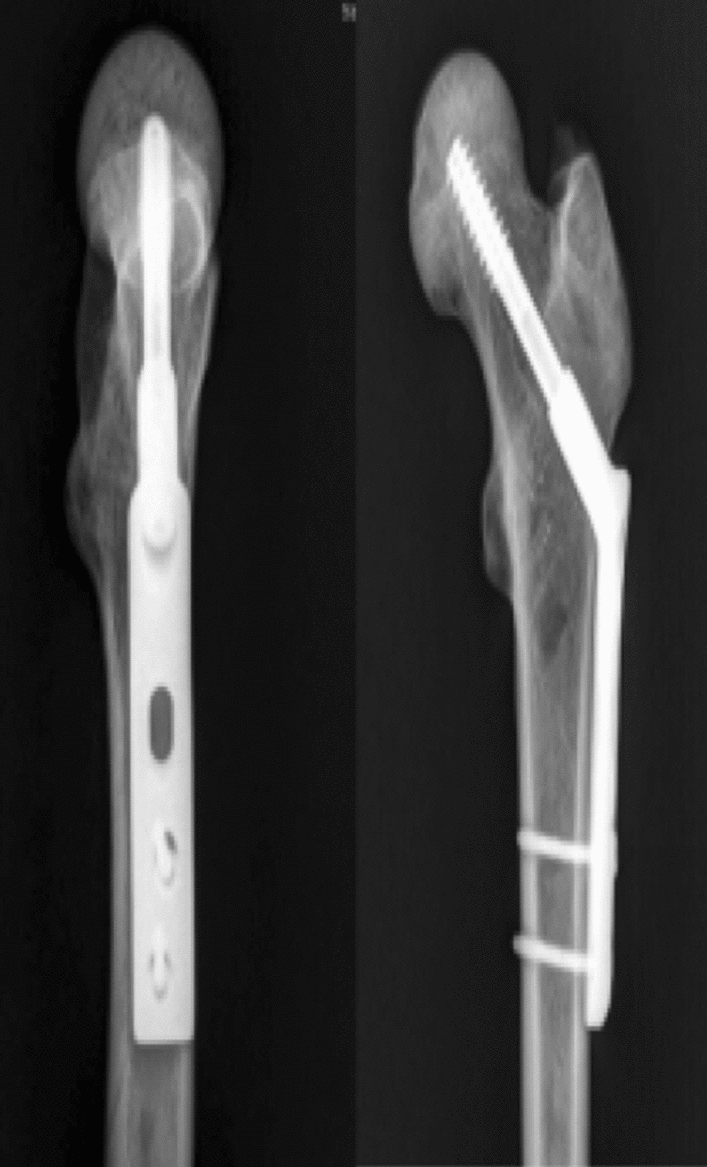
Dynamic hip screw (DHS) (*Note*: Positive and lateral X-ray film after internal fixation).
